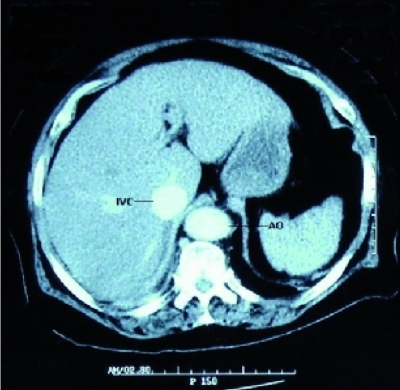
Abdominal CT scan showing simultaneous contrast enhancement in the aorta and IVC
